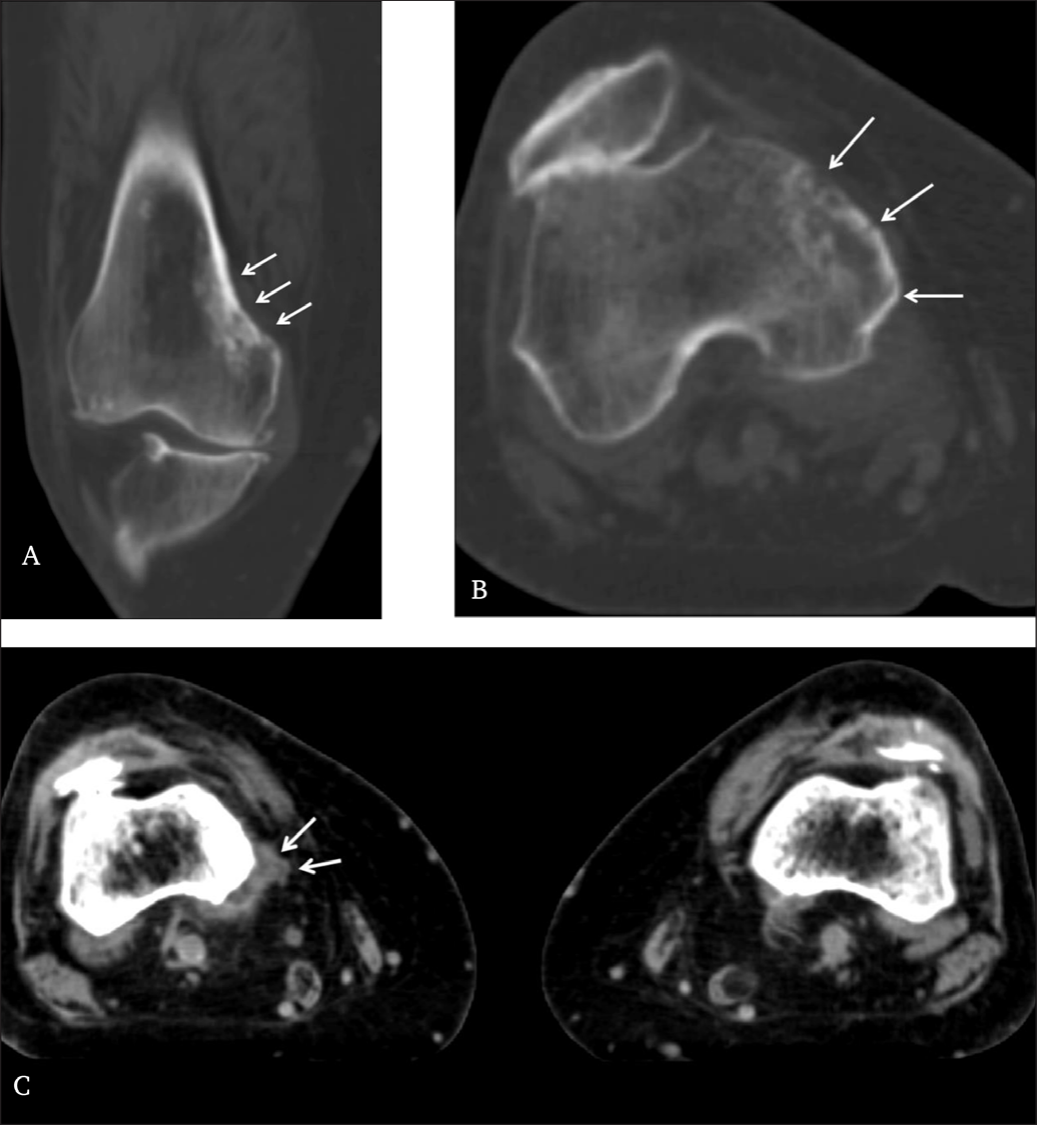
A. Coronal reconstructed CT image and B. axial CT image through distal right femur in bone window show the extent of the cortical metastases and underlying sclerosis more clearly (arrows). C. Axial CT scan through distal femur in soft tissue window shows a discrete associated soft tissue mass posteromedially on the right side, compared with the normal left side (arrows).
